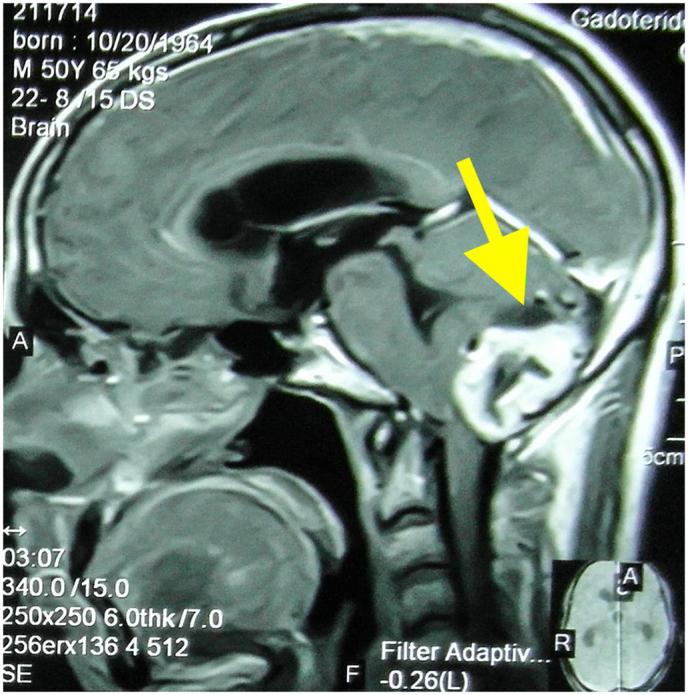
T1-weighted postcontrast MRI showing cerebellar hemangioblastoma (arrow).
